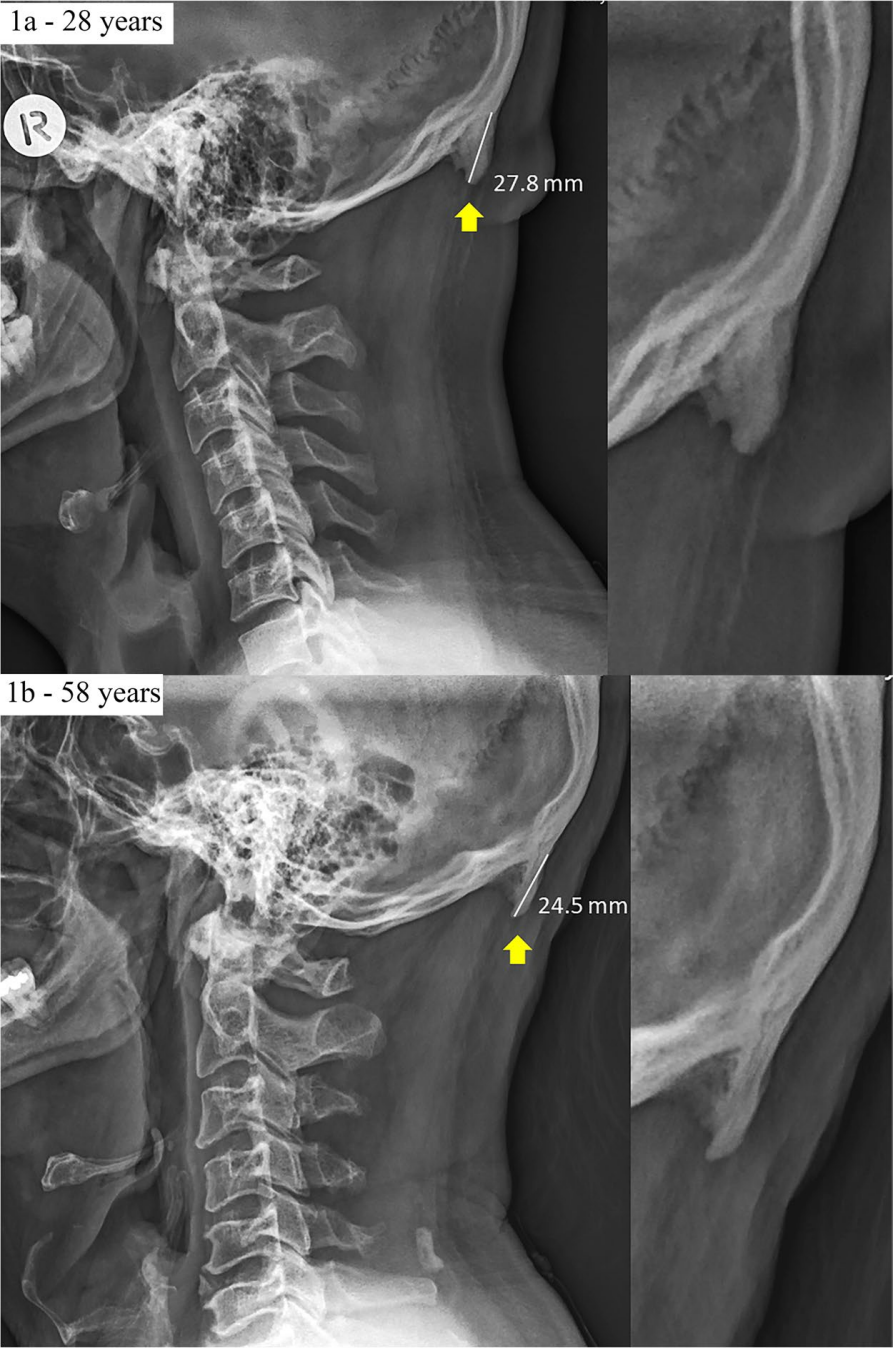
Example radiographs of two male participants (28-years-old and 58-years-old) presenting with large enthesophytes emanating from the occipital squama. These images also include the enthesophyte measurements used throughout this study.
